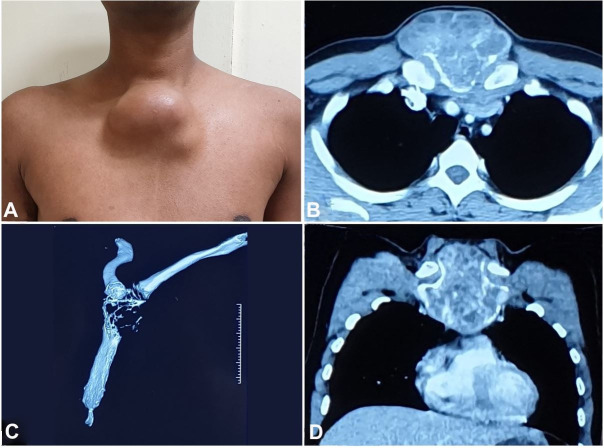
A – Clinical photograph of the patient showing a lobulated swelling over the manubrium sterni; B-D – Contrast-enhanced computed tomography showing expansile lytic lesion involving manubrium sterni and proximal body of sternum with large heterogeneously enhancing soft tissue component, peripheral rim of calcification and contiguous infiltration of adjacent pectoralis major (B – Axial plane; C – Sternal 3D reconstruction; D – Coronal plane).
